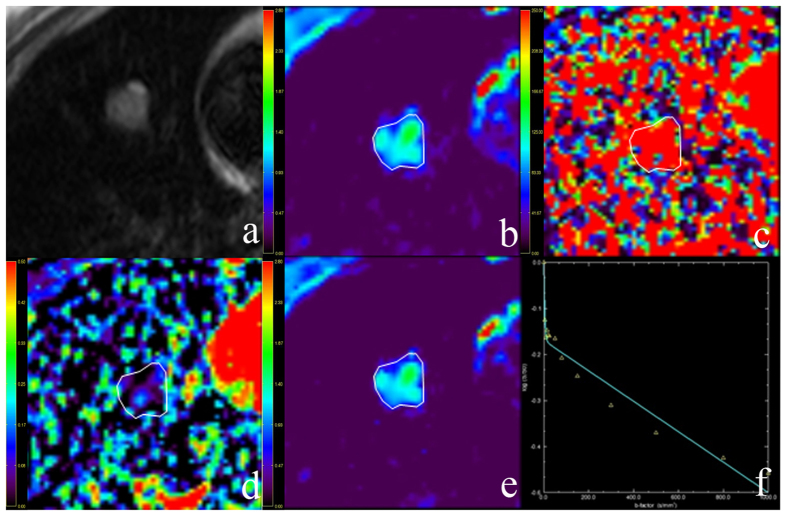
Representative imaging characteristics in a 38-year-old woman with right middle lobe benign sclerosing pneumocytoma. (a) T2-weighted image. (b) The D map. (c) The D* map. (d) The f map. (e) Apparent diffusion coefficient (ADC) map. (f) The bi-exponential fitting of the diffusion signal decay. The tumor shows slight increase on T2 signal intensity and slightly high signal on D and ADC maps (encoded in cyan and green colors), which indicates a benign tumor. The f map demonstrates a relatively low perfusion fraction region compared to the lung. The D* map shows a high pseudo-diffusion values in the tumor tissue.
